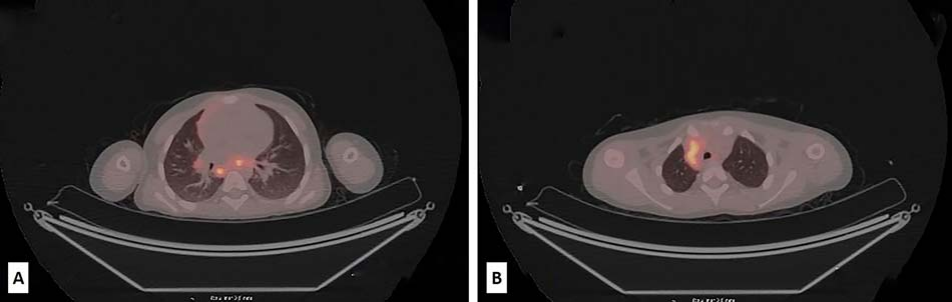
Low-dose PET/CT scan from the level of upper thoracic aperture revealing enlarged and metabolically active retrocaval and mediastinal lymph nodes, with SUV_max_ 8.6 and SUV_max_ up to 6.5 (A). Also, at the level of the carina and subcarinal region metabolically active lymph nodes were seen (SUV_max_ up to 7.6). In the right hilus, metabolically active lymph node SUV_max_ up to 3.5 was also observed (B).
